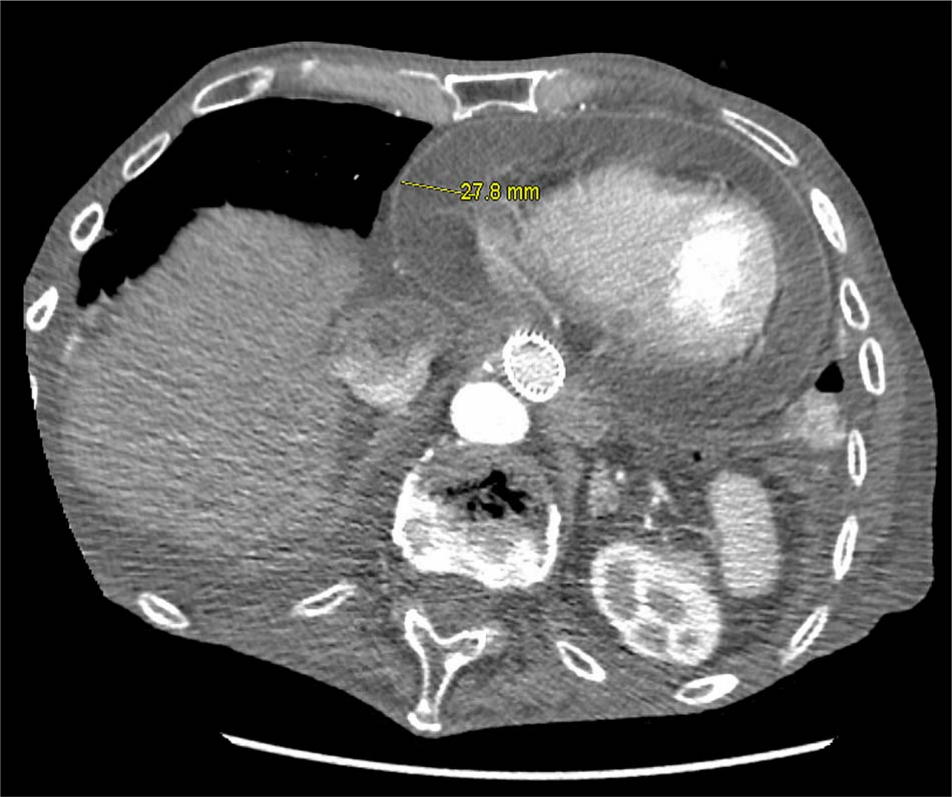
CT scan of the chest showing a large pericardial effusion with maximal thickness of 27.8 mm.
